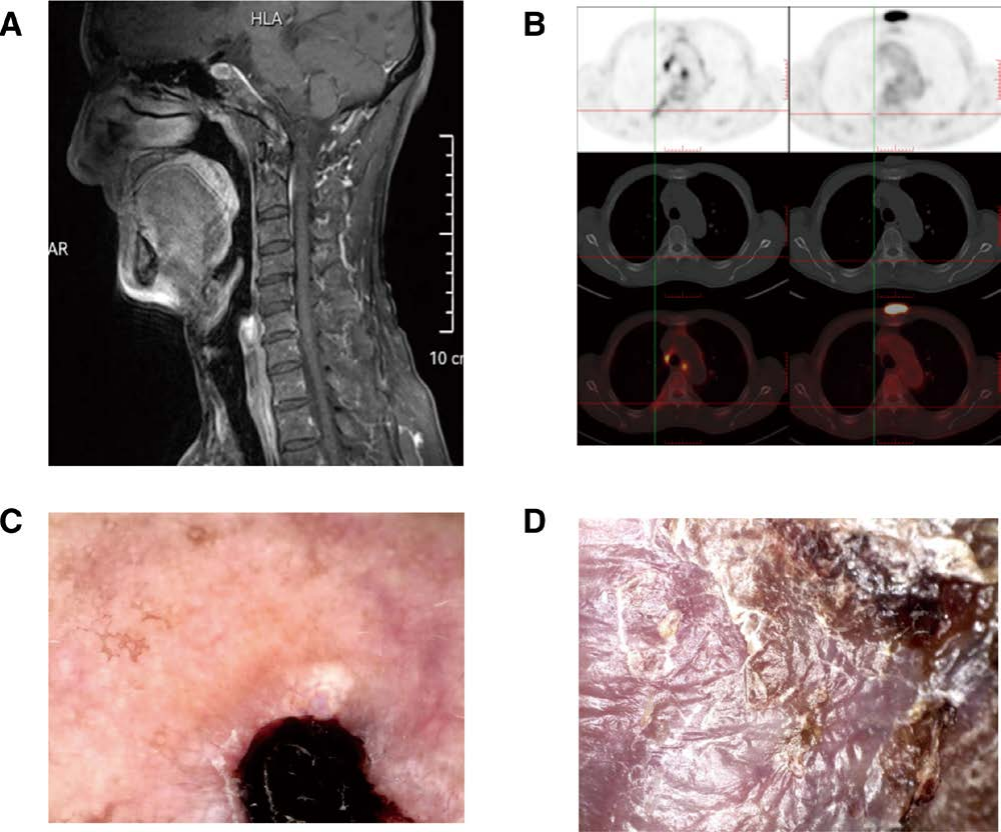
(A) Longitudinal nasopharyngeal MR scan: a slight thickening of the skin at the tip of the nose (B) Chest transverse PET-CT scan: anterior chest wall occupying with increased FDG metabolism (SUV max = 11.4) (C) Dermoscopic image of the nose: a well-defined blue-gray mass on nose with slender, branched blood vessels. (D) Dermoscopic image of the chest: a white or yellow unstructured area on a red background.
